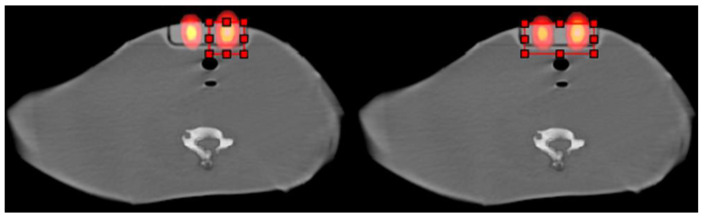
ROIs (red outline with dots) around (left) one and (right) both volumes on the SPECT/CT fused middle slice.
